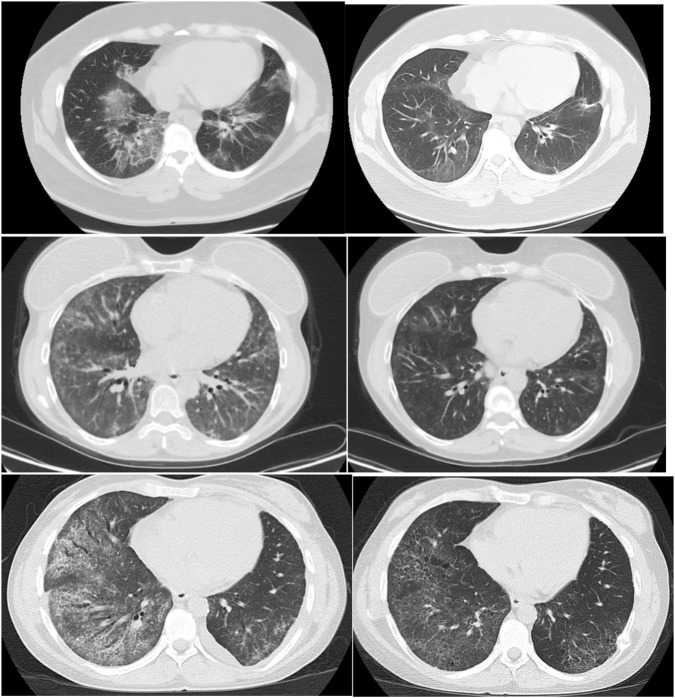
CT scan findings before (left) and after (right) rituximab therapy showing improvement in three of the nine patients who had repeat CT chests within 12 months of rituximab therapy.
